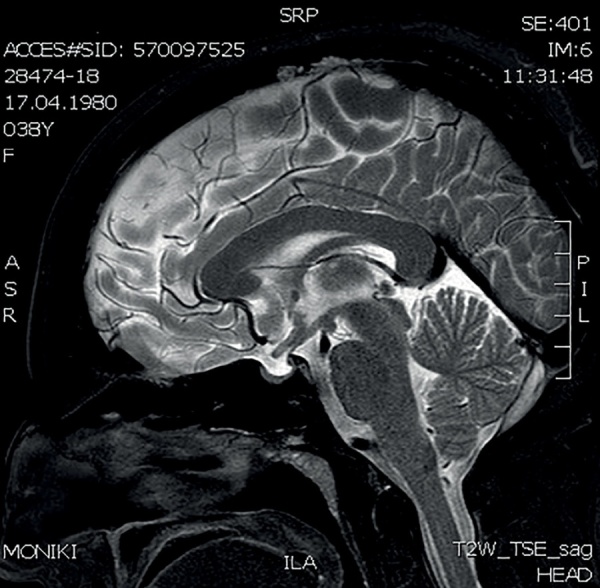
Рисунок 2. МРТ головного мозга с к/у от 24.11.2017г (3 Тл)МР-картина «пустого» турецкого седла, ранее выявленное образование в гипоталамо-гипофизарной области не определяется. Figure 2. MRI of the brain with an appointment dated November 24, 2017 (3 T)MRI picture of an “empty” sella turcica, a previously identified formation in the hypothalamic-pituitary region is not detected.
